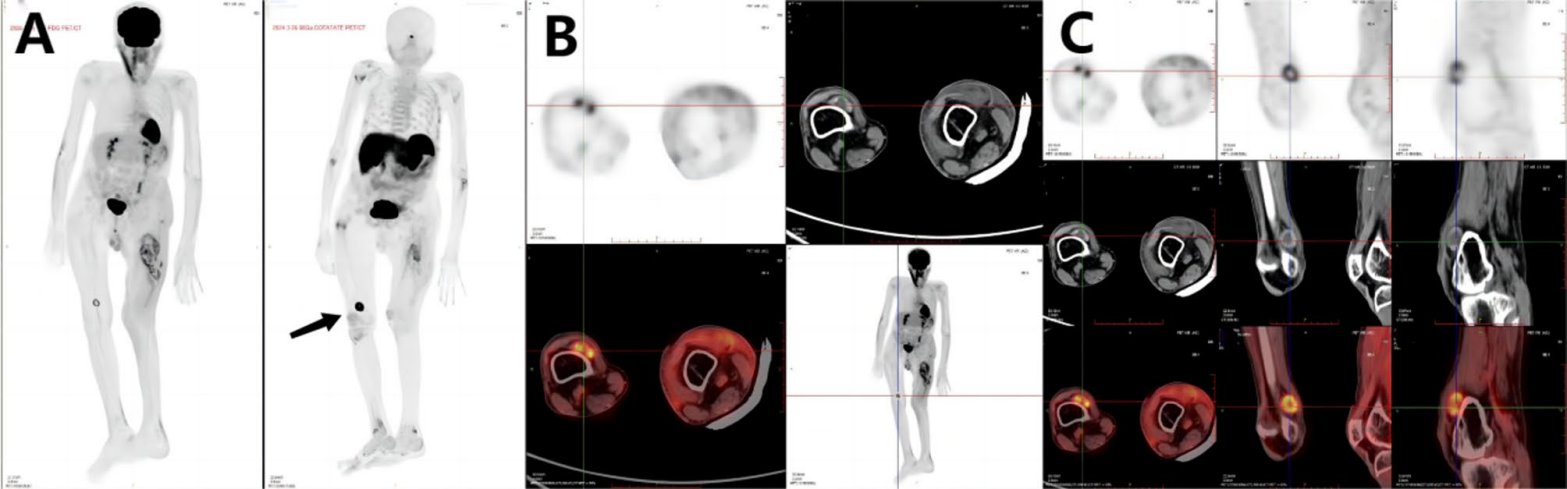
Complete body and tumor localization images from 68 Ga-DOTA-TATE PET/CT
